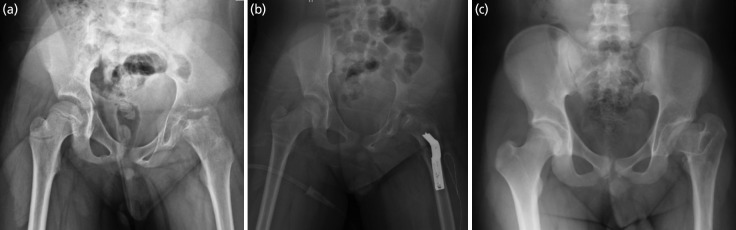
The series of radiographs belongs to patient no.5. (a) The presentation at the age of 10 showed fragmentation phase with Herring C. (b) The immediate post-surgery showed good containment. (c) The hip at the age of 17 years old showed Stulberg III.
